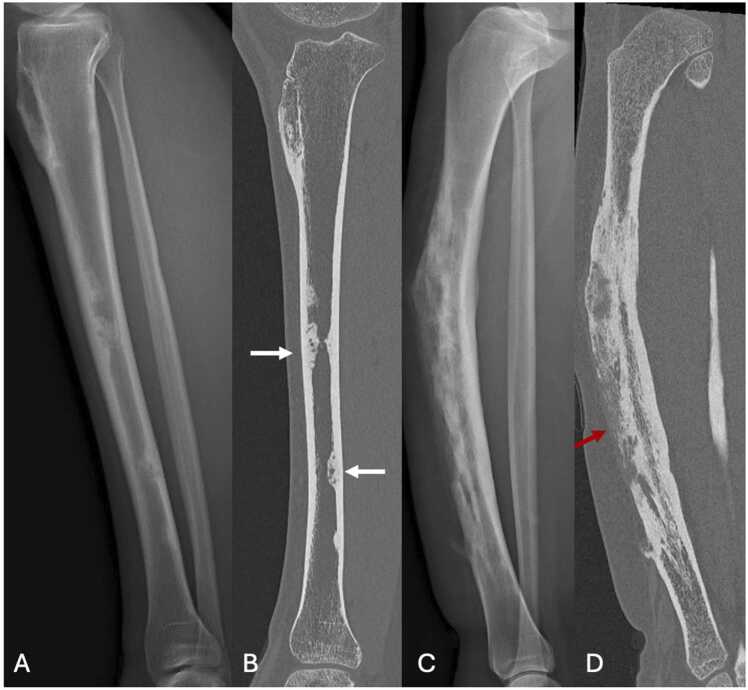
A,B. Plurifocal osteofibrous dysplasia of the left tibia in a 19-year-old young woman. C,D. Adamantinoma of the right tibia in a 14-year-old girl (different patient). A. Profile radiography showing plurifocal sclerotic lesions of diaphyseal cortical of the tibia. B. UHR CT in sagittal plane highlighting those sclerotic lesions with central hypodense areas, no cortical destruction and endosteal extension along the long axis of the diaphysis (white arrow). C. Profile radiography showing a large ill-defined lesion of the middle diaphysis of the tibia, with cortical thickening and multiple lacunae of the anterior cortical bone producing a « soap-bubble » appearance. Note the curvature of the tibia. D. UHR CT in sagittal plane highlighting cortical destruction of the anterior cortical bone (red arrow) and involvement of the medullar cavity.
